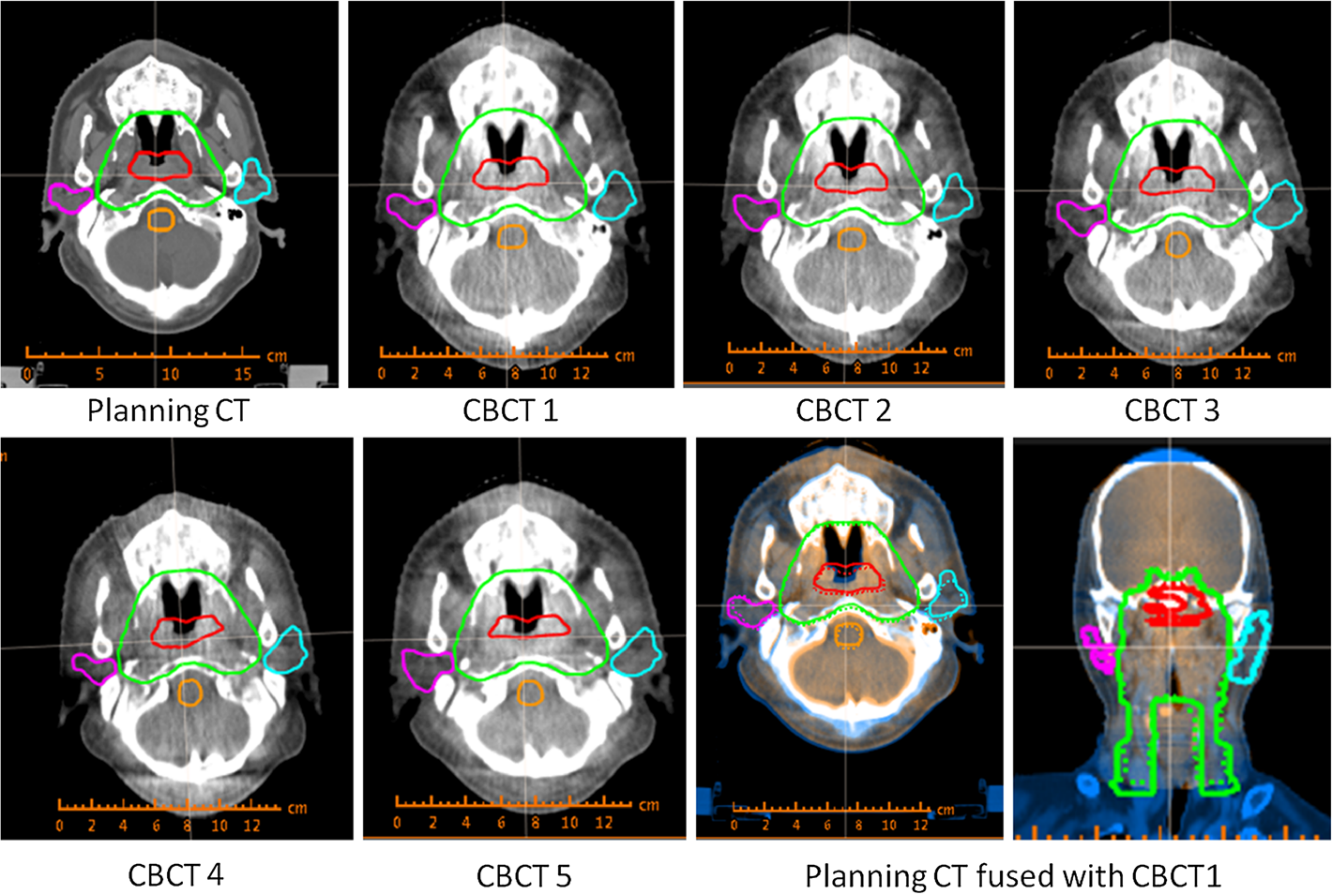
Planning CT with manual contours, CBCTs with deformed contours and a typical fusion image.
